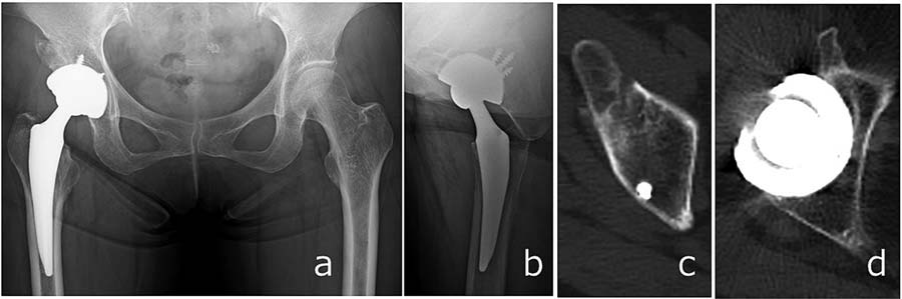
Radiographs (a and b) and CT images (c and d) obtained at 6 months postoperatively, showing stable implant fixation and partial union of the anterior inferior iliac spine and acetabular anterior wall fractures.
